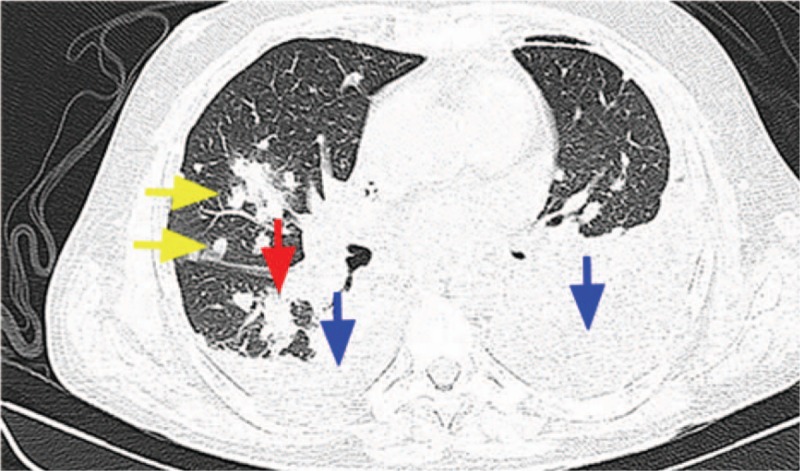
Chest computed tomography of the patient showing multiple pulmonary nodules (the yellow arrow), partial consolidation (the red arrow), and bilateral pleural effusion (the blue arrow). *Burkholderia pseudomallei* was isolated from a culture of pleural empyema, blood cultures, tracheal aspirate, and urine.
